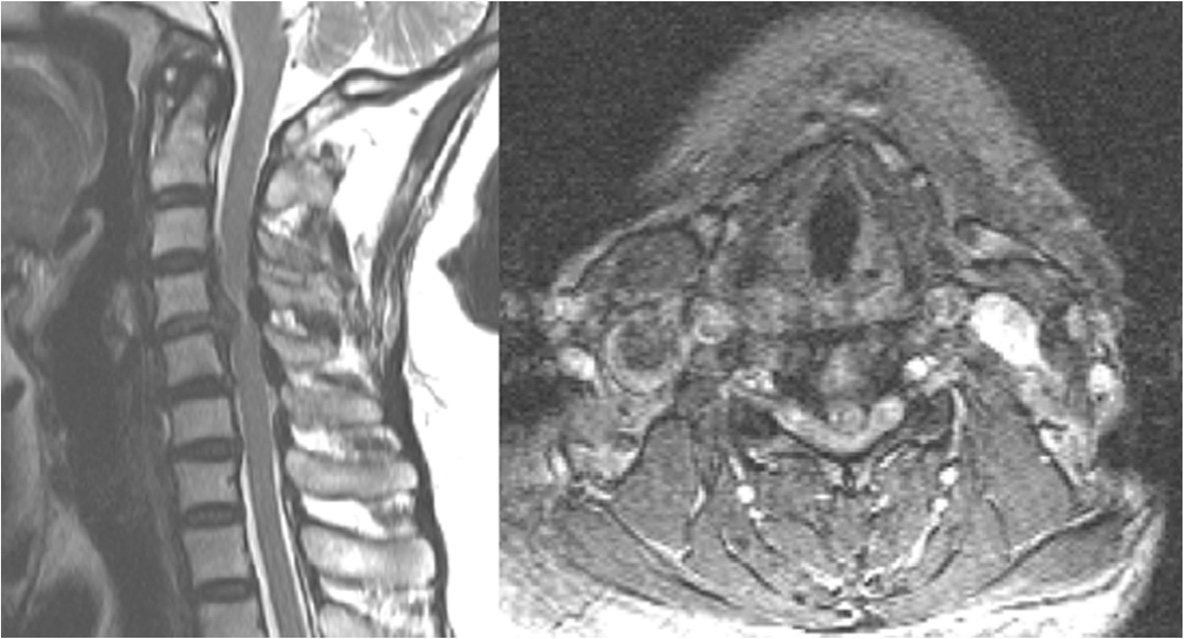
Sagital T2 magnetic resonance image of a typical study patient showing single-level cervical spondylotic myelopathy (C4-5)
